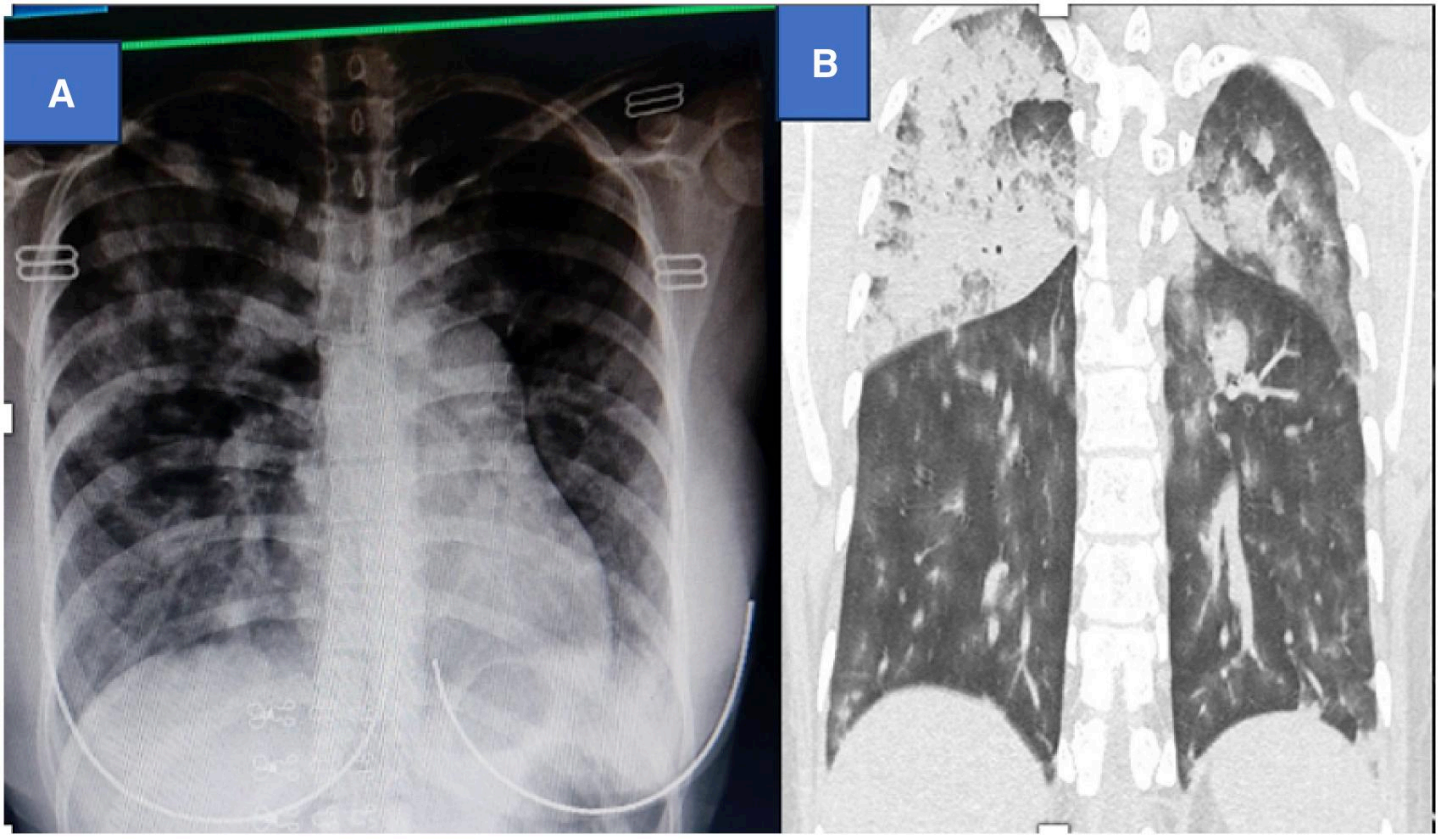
Chest X-ray showed diffuse patchy airspace opacities in the bilateral lung field (Figure A). Figure (B) coronal HRCT showed confluent ground glass opacities with air bronchogram in bilateral upper lobe (right > left); predominant air space opacities in bilateral upper lobe of lung.
